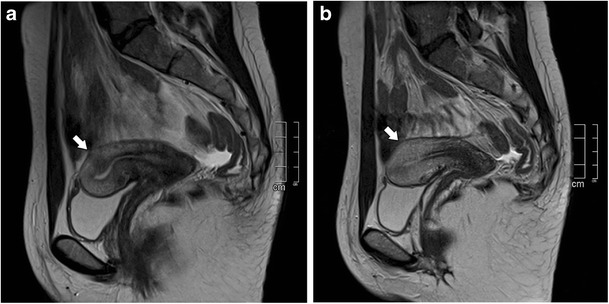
Uterine contractions mimicking adenomyosis: a and b Sagittal T2-weighted images; hypointense bands perpendicular to the junctional zone that modify after a few minutes (white arrows), and representing physiologic uterine contractions
